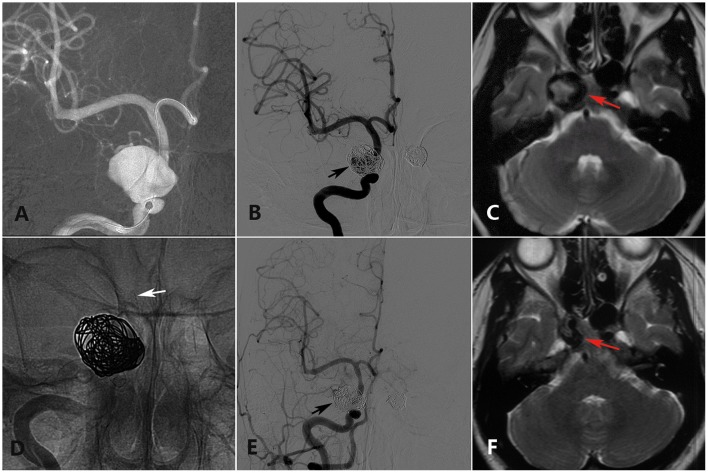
A 28-year-old woman with a double cavernous segment ICA aneurysm (the left-side aneurysm was excluded from analysis) presented with incomplete ONP of the right side showing full recovery in the last clinical follow-up. (A) Preoperative DSA shows a giant cavernous segment ICA aneurysm. (B) Immediate postoperative DSA shows loose embolization of the right aneurysm (black arrow). (C) Preoperative axial-plane MRI shows a cavernous segment ICA aneurysm (red arrow). (D) Intraoperative DSA shows placement of a PED (white arrow) covering the aneurysmal neck. (E) Twelve-month DSA shows collapse of the coils in the sac. (F) Twenty-four-month axial-plane MRI shows shrinkage of the aneurysm (red arrow).
